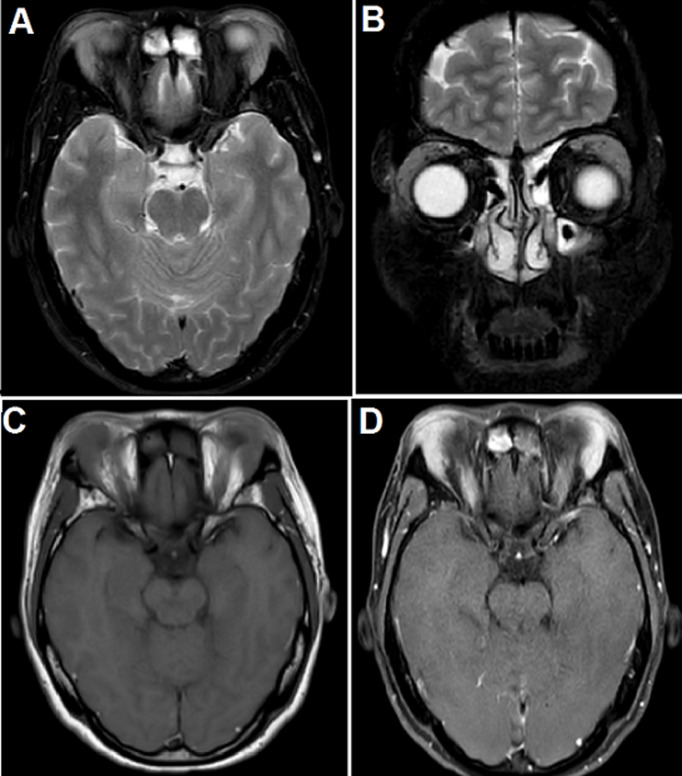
MRI showed enlarged lacrimal glands before treatment: A, B) fat-suppressed T2-weighted images demonstrated the hypointense bilateral swelling of the lacrimal glands; C) the lacrimal glands were isointense to skeletal muscle on T1-weighted images; D) T1-weighted images post-contrast showed that the lacrimal glands are remarkably and homogeneously enhanced
